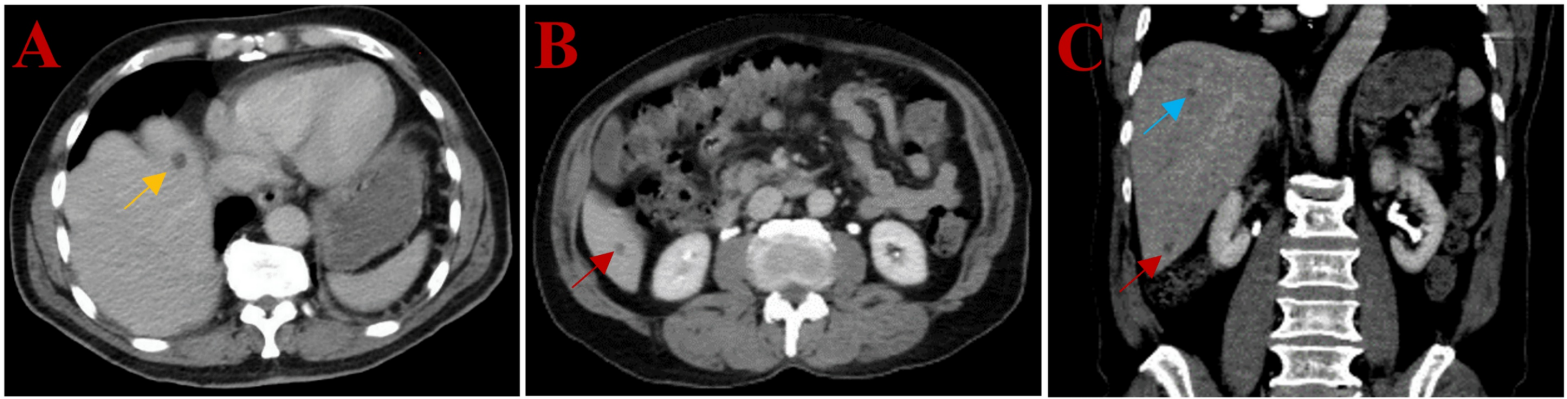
Computed tomography imaging A) CT scan of the abdomen without contrast, axial view demonstrating left hepatic lobe hypoattenuation measuring 1.1 cm in largest axis diameter (yellow arrow); B) CT scan of the abdomen without contrast, axial view demonstrating right hepatic lobe hypoattenuation near the inferior hepatic border (red arrow); C) CT scan of the abdomen without contrast, coronal view demonstrating hypoattenuations in the right lobe (blue and red arrows) CT: Computed tomography
